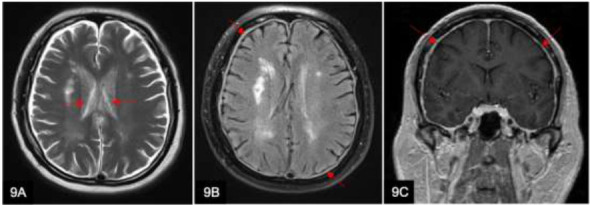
A 70-year-old woman with a past history of intracranial hemorrhage underwent ventriculostomy for hydrocephalus. Post-operative Axial T2w (A), axial FLAIR (B), and coronal T1w post-contrast MRI brain scan (C) show slit-like ventricles (A arrows) and diffuse pachymeningeal thickening and enhancement (B and C arrows) due to intracranial hypotension related to chronic shunting.
